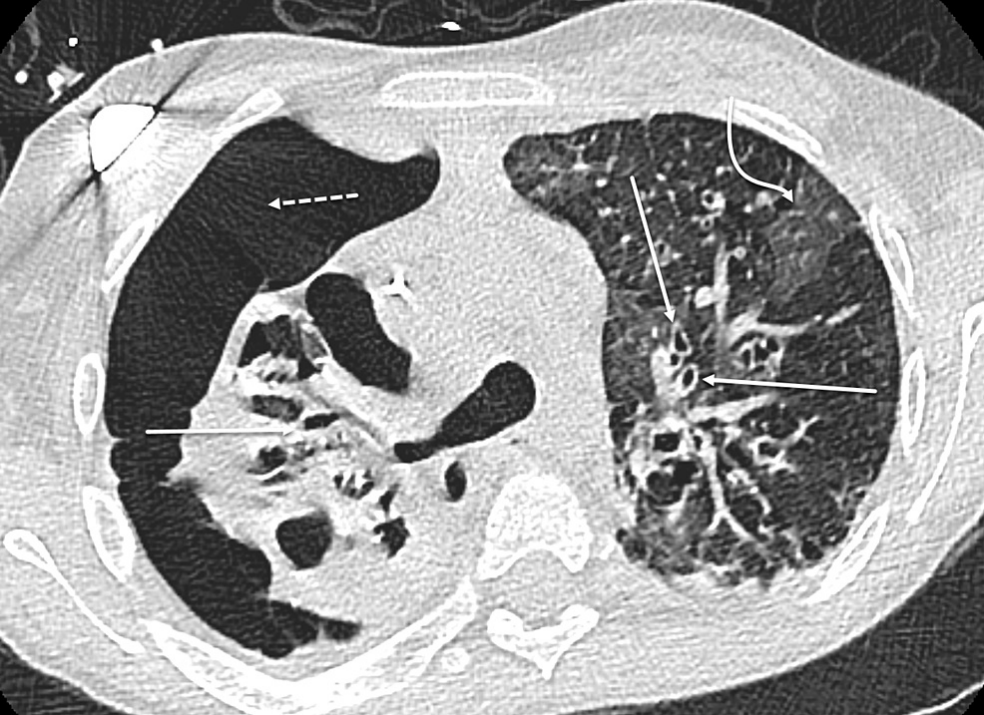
CT image chest without contrast. Multiseptated large right pneumothorax (dotted arrow), septations not shown. Advanced findings of CF with bilateral bronchiectasis (solid arrows) and concomitant ground glass infection (curved arrow). CF, cystic fibrosis
